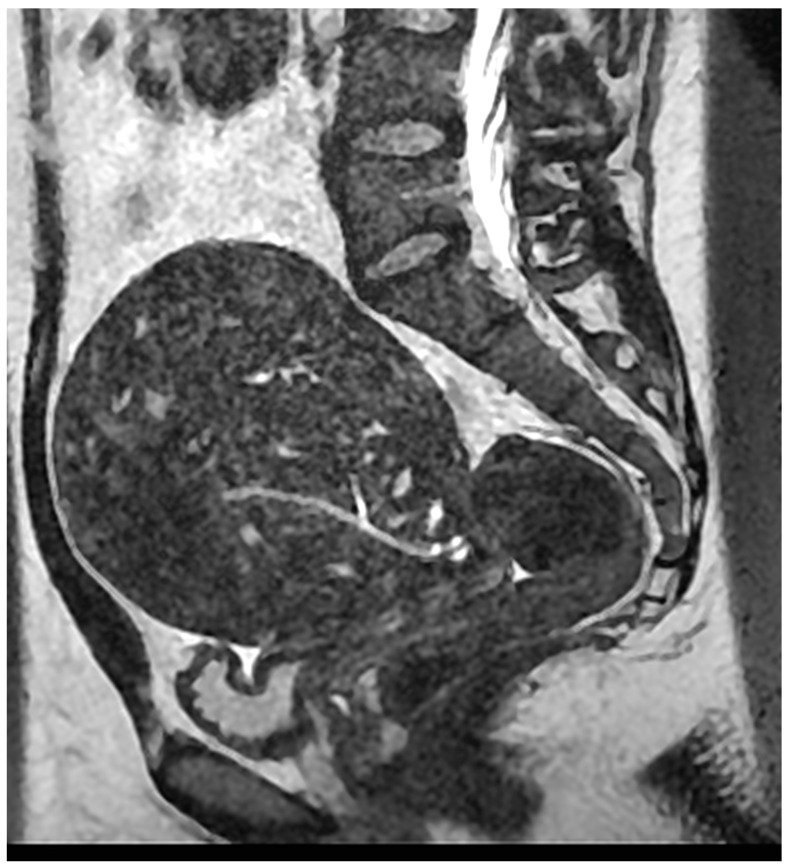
Magnetic resonance imaging (MRI) sagittal T2-weighted MRI showing a globular uterus containing multiple endometrial foci related to deep diffuse internal adenomyosis. Reproduced with permission from Habiba et al. (2020).
